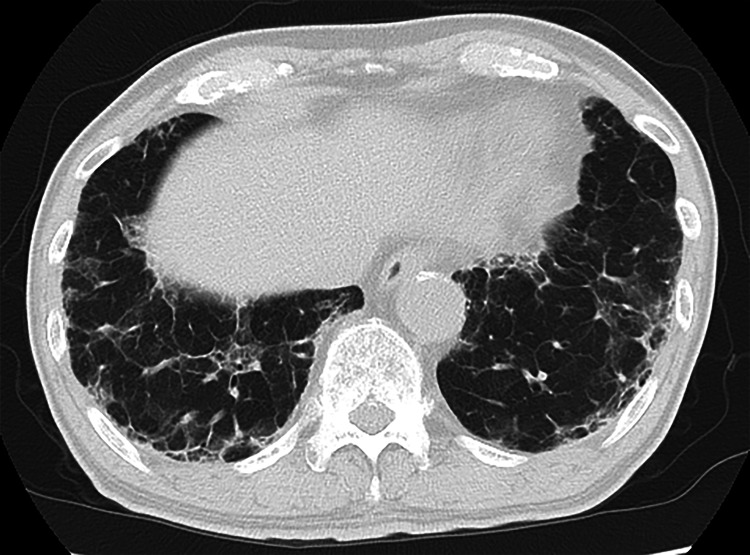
High-resolution lung CT scans show bilateral subpleural and peribronchovascular ground-glass opacities and reticular lesions with traction bronchiectasis, suggesting an NSIP pattern. NSIP: Non-specific interstitial pneumonia
